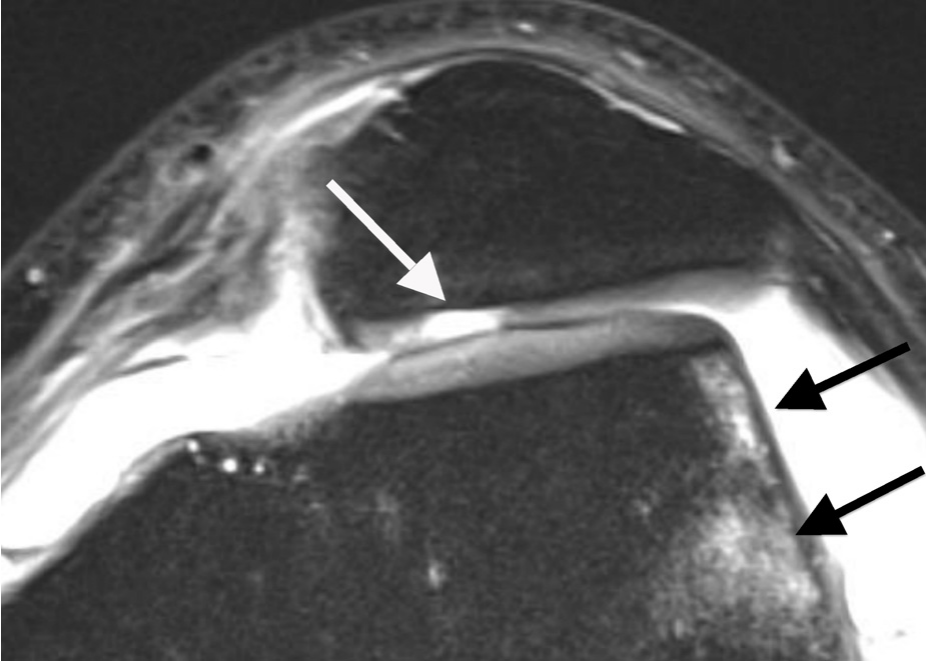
Axial PD-weighted TSE MRI of a 28-year-old male with a first LPD. MRI shows bone marrow edema involving the anterolateral femoral condyle (black arrow). At the central dome of the retropatellar articular surface, a full-thickness defect of the cartilage with denudation of the bone is visible. This finding is defined as a grade 4 disorder (white arrow).
